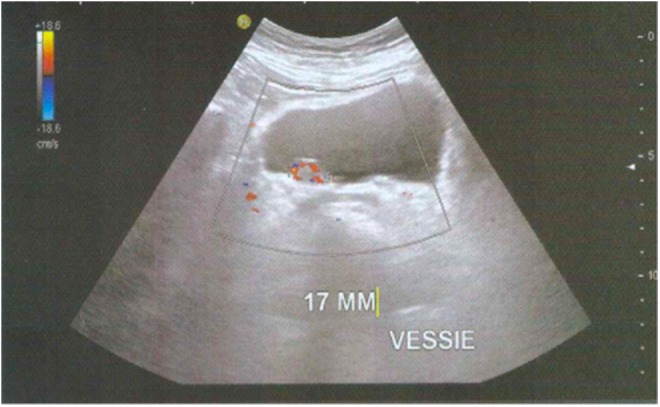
Ultra sonogram of the urinary bladder showing a bladder nodule.
